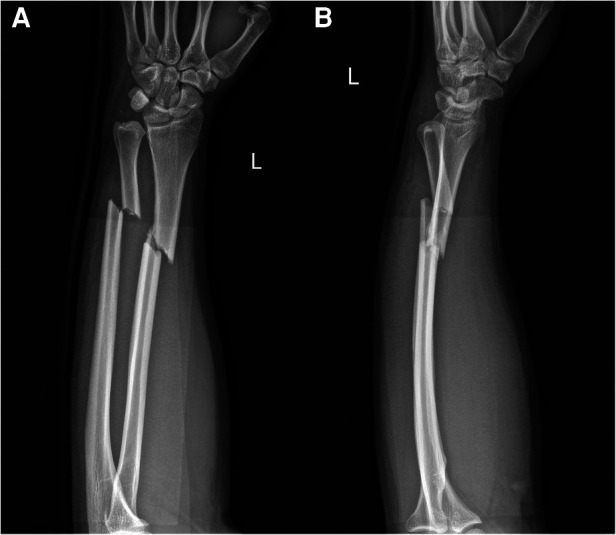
X-ray images (A,B) of the left forearm showed both bone forearm fractures with marrow edema.
